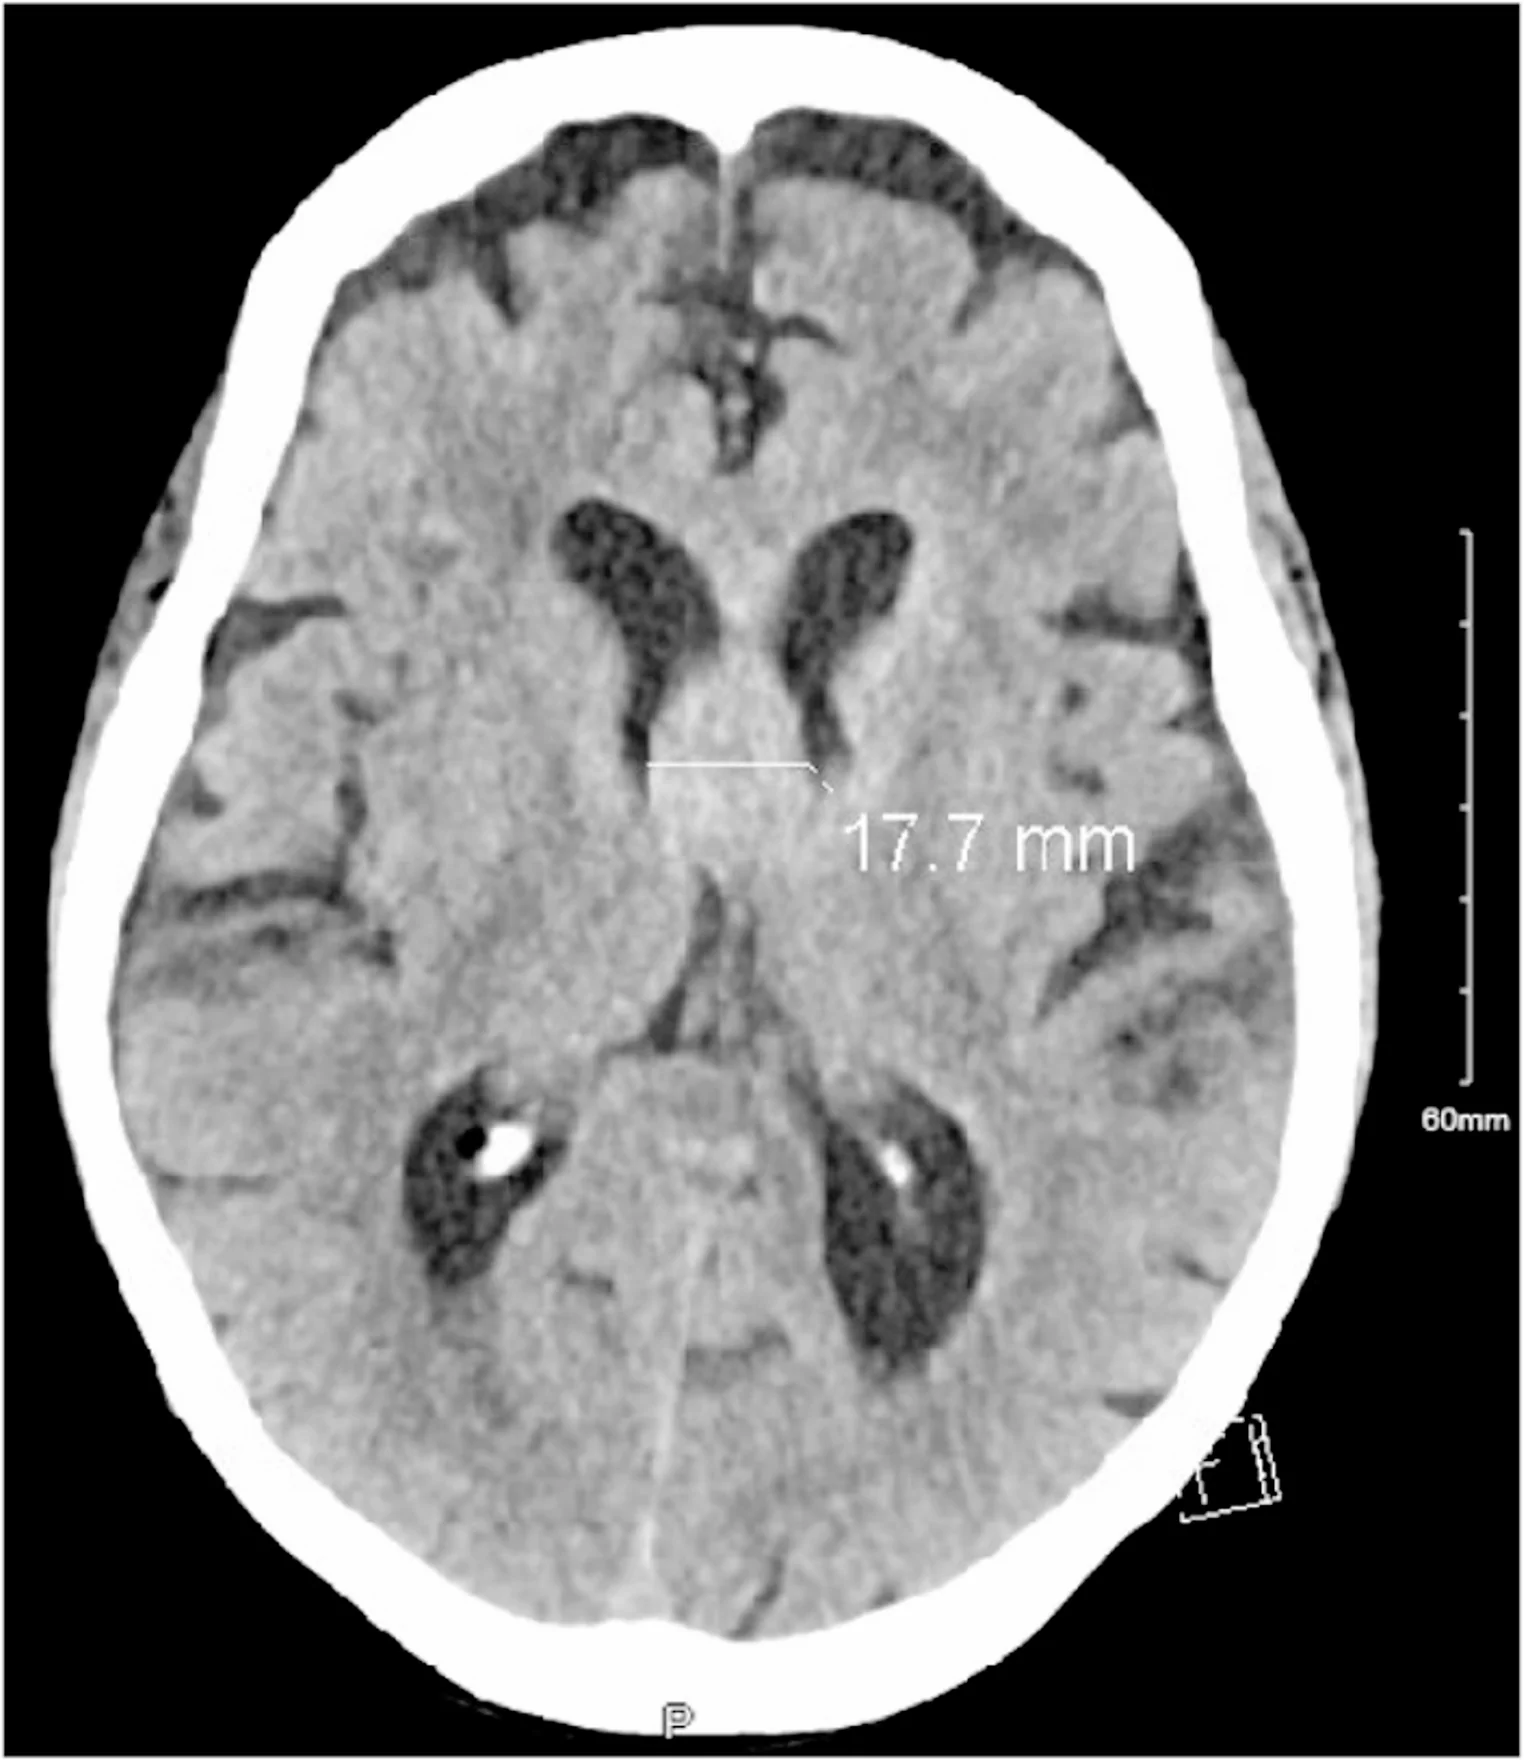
Axial view of a simple computed tomography of the brain showing diffuse thickening of the interventricular septum without hydrocephalus
